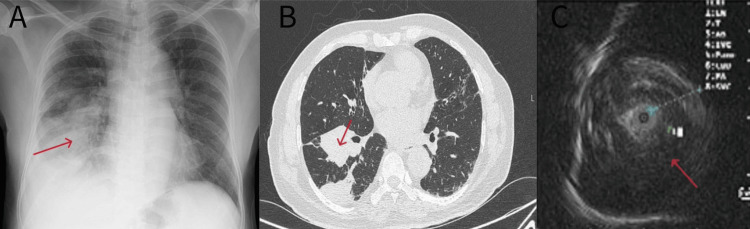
(A) Chest X-ray showing consolidation in the right lower lobe (arrow). (B) High-resolution CT of the thorax demonstrating evidence of subpleural and peribronchial consolidation in the right lower lobe (arrow). (C) R-EBUS image demonstrating central hypoechoic mass with linear arcs and dots within the lesion (arrow), surrounded by a hyperechoic margin, thus confirming R-EBUS within the lesion. CT: computed tomography; R-EBUS: radial endobronchial ultrasound
